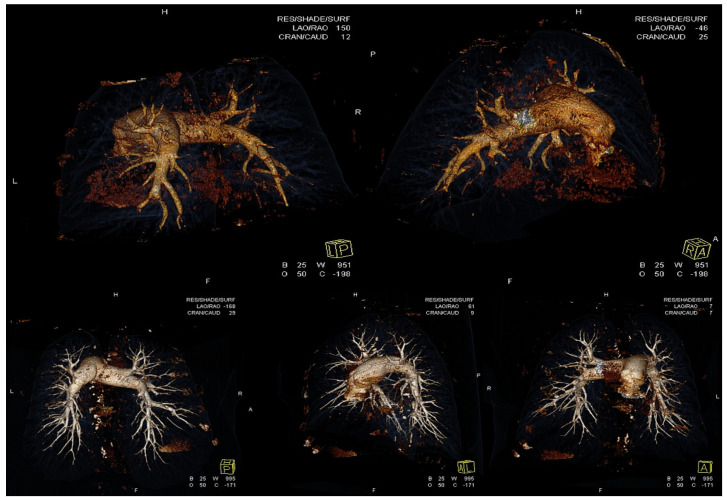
The improvement of anatomy in the pulmonary arterial tree via a Computed Tomography Angiography/3-D reconstruction study in a patient treated with BPA. Upper panel shows the pulmonary artery tree before BPA. Right and left main pulmonary arteries where some lobar and segmental branches are visible but without subsegmental branches. Lower panel shows the pulmonary artery tree after BPA where the majority of segmental and subsegmental branches are recanalized.
